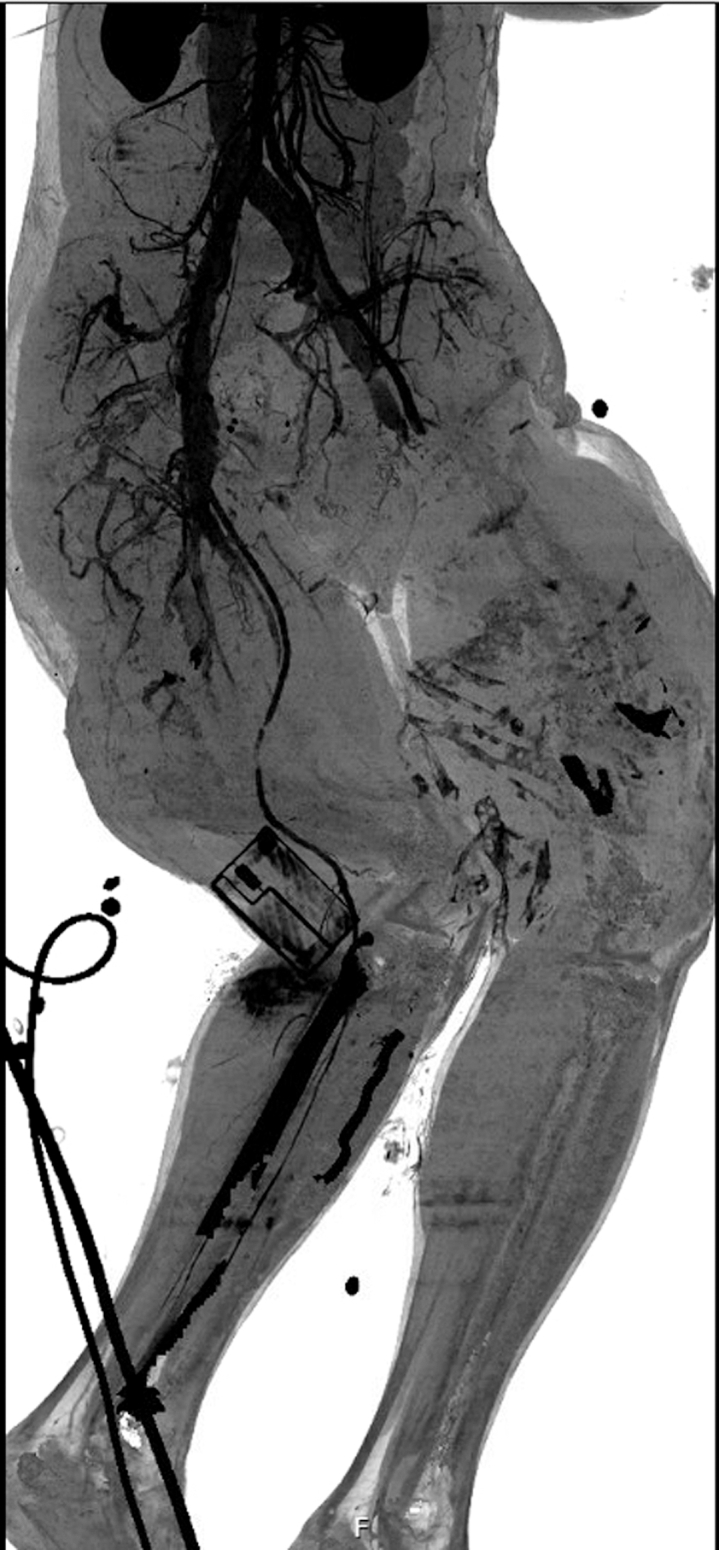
Initial computed tomography angiogram demonstrating abrupt loss of contrast opacification in the left superficial femoral (SFA) and popliteal arteries, consistent with complete vascular transection. This image confirms that the injury extended beyond the SFA into the popliteal segment, supporting the need for long-segment endovascular repair.
